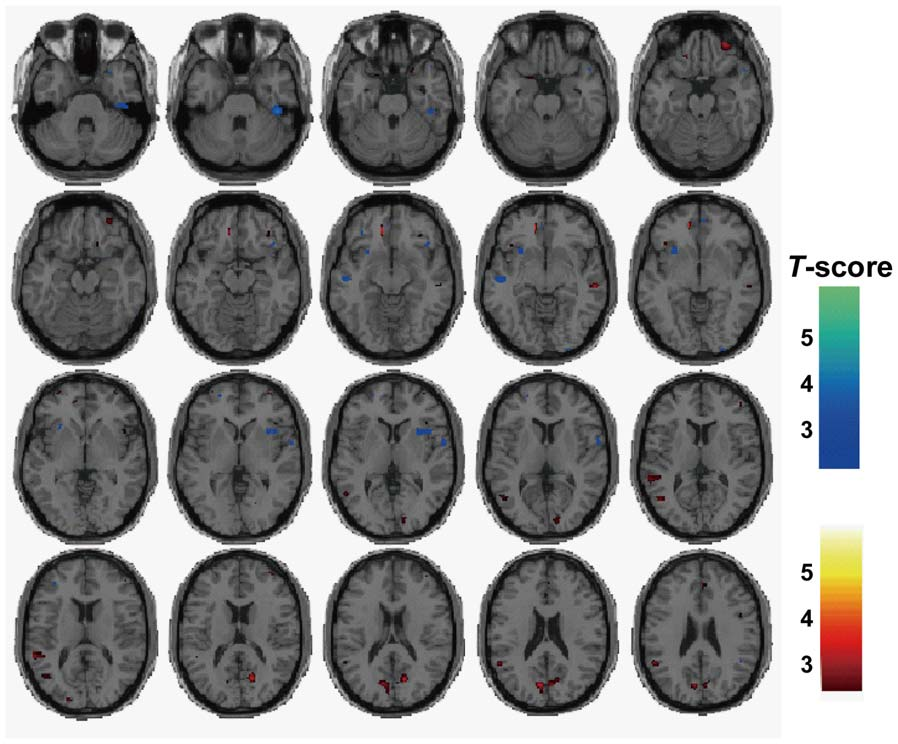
Effects of the risk-T-allele on decreased GM regions and diagnosis-*NRGN* genotype interaction on GM regions. Effects of the risk T allele on decreased GM regions (TT<CT<CC) in total subjects were shown by whinter colormap (blue areas). Diagnosis-*NRGN* genotype interaction on GM regions was shown by hot colormap (red areas). There was no significant effect of the risk T allele on increased GM regions (CC<CT<TT) among the total subjects. Each colormap shows *t* values corresponding to the color in the figure.
